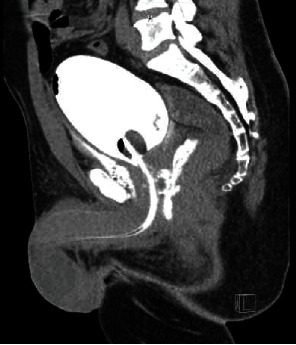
Case 3: sagittal CT cystogram showing extraperitoneal contrast extending from the prostatic urethra.
